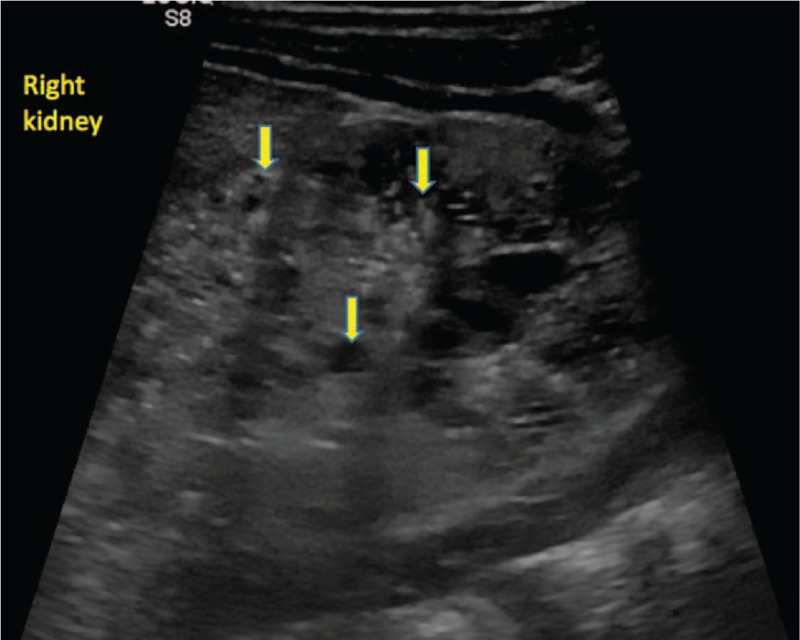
The evolution of cystic lesions in the right kidney (Ultrasound aspect - 1 year and 2 months of age).
